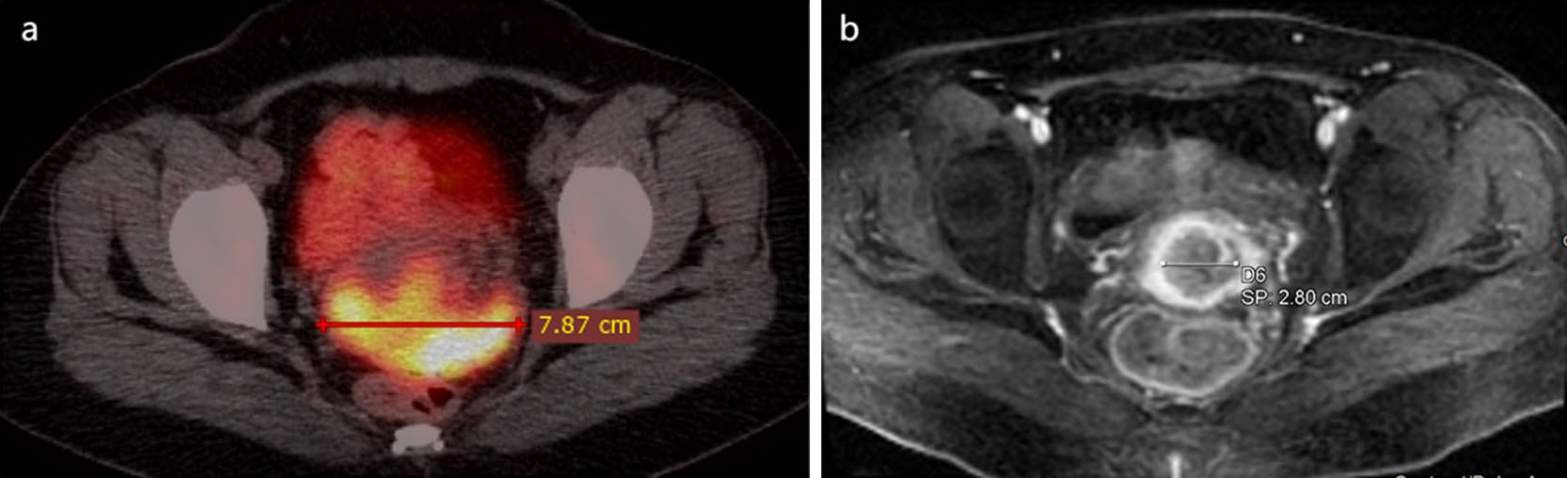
Images of a 31-year-old patient with (FIGO 2018) stage IIIC squamous cell carcinoma before and after treatment. (a) Axial fusion PET/CT images of pelvic before treatment. (b) Axial T2WI MRI image of pelvic 3 months after treatment.
